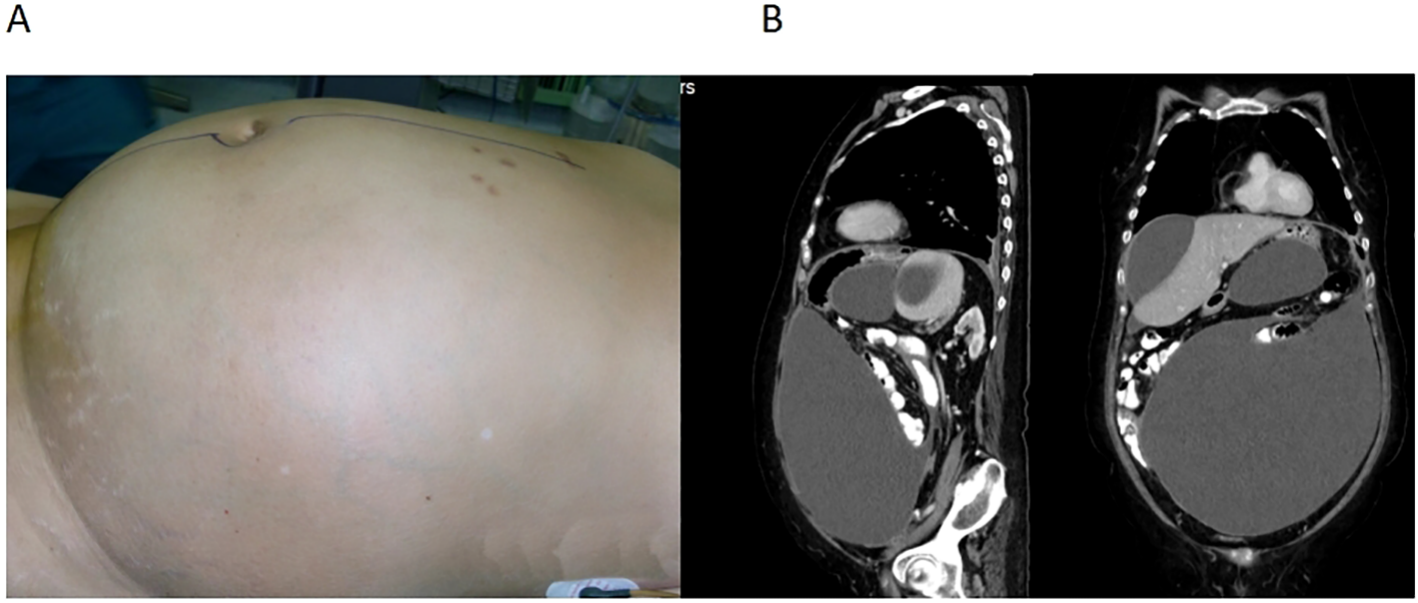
(A) A large abdominal circumference of 121 cm. (B) Computed tomography showing cystic solid nodule in the right adnexal area, diffuse thickening of the peritoneum and greater omentum, and multiple encapsulated fluid accumulation in the abdominal and pelvic cavities.
